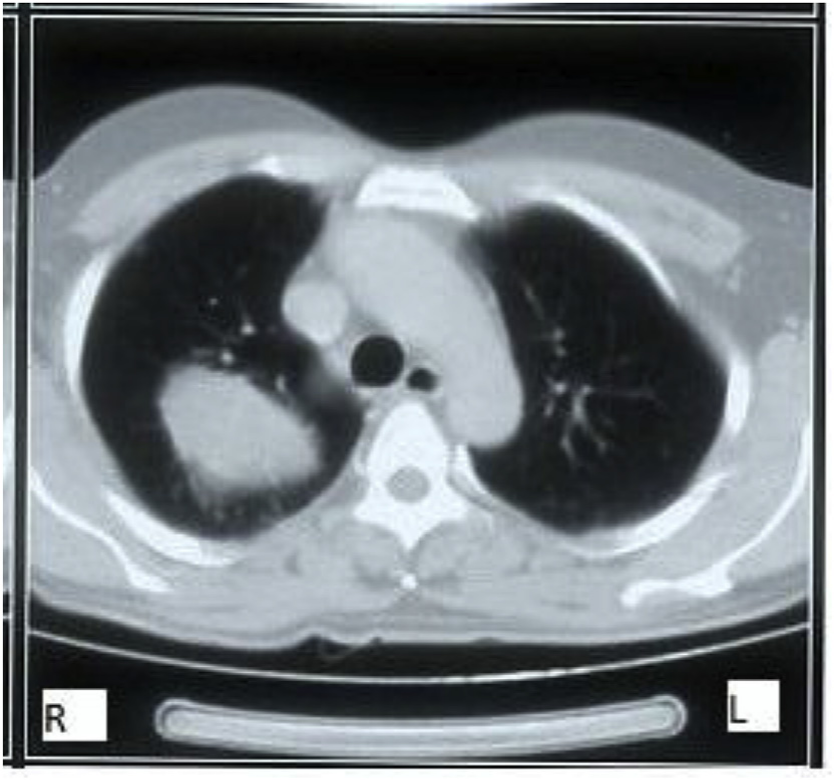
CT scan chest showing a heterogenously enhancing lesion in right upper lobe.
